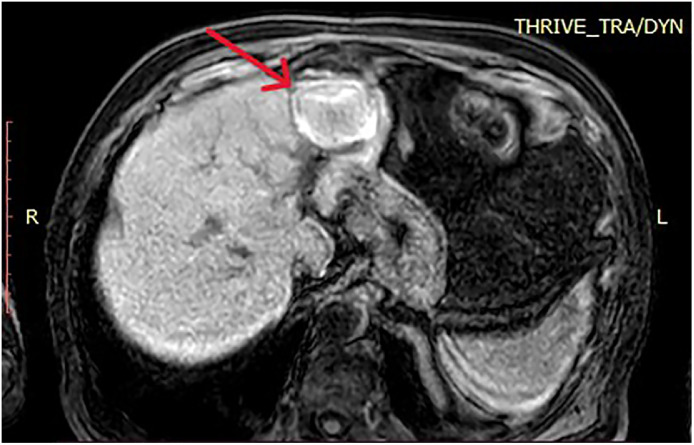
One-month follow-up MRI (Post-contrast T1-weighted) showing complete technical success with a non-enhancing ablation zone (red arrow) and total resolution of hemopericardium.
